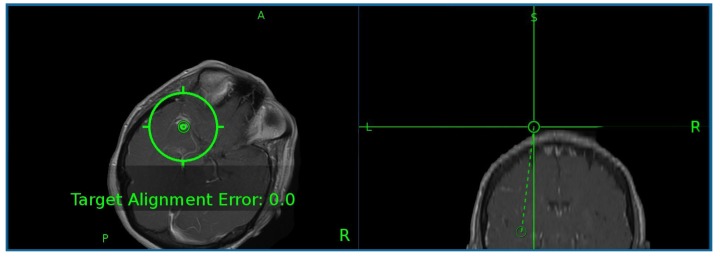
Intraoperative magnetic resonance imaging pinpointing focus of maximal enhancement for stereotactic biopsy planning.
